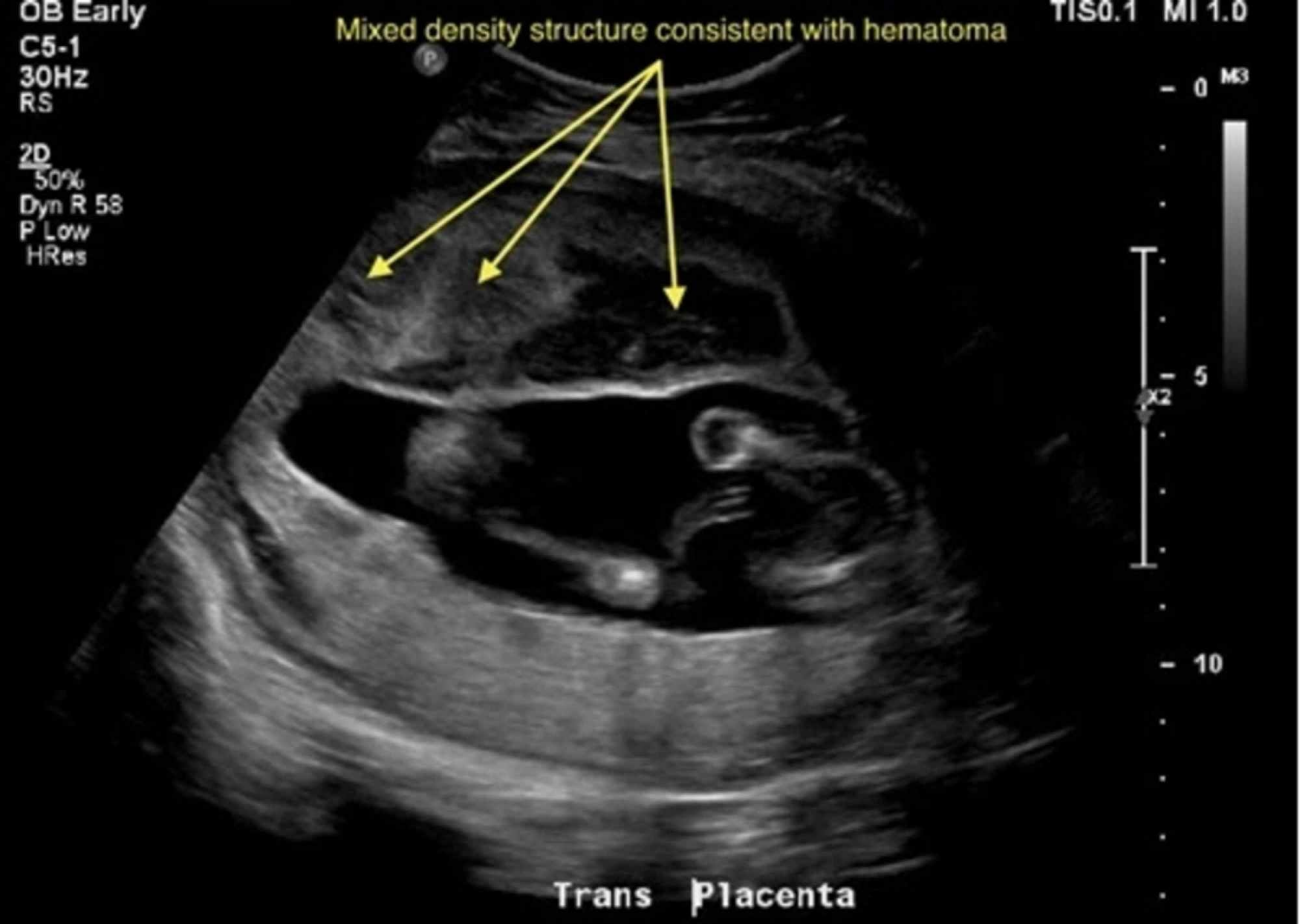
"U/S OB trauma limited" post motor vehicle collision, showing notable anterior echogenic material, which appears heterogeneous in echotexture with hypoechoic areas measuring 12.0 x 5.4 x 9.5 cm U/S, ultrasound; OB, obstetric; findings were concerning for placental abruption.
